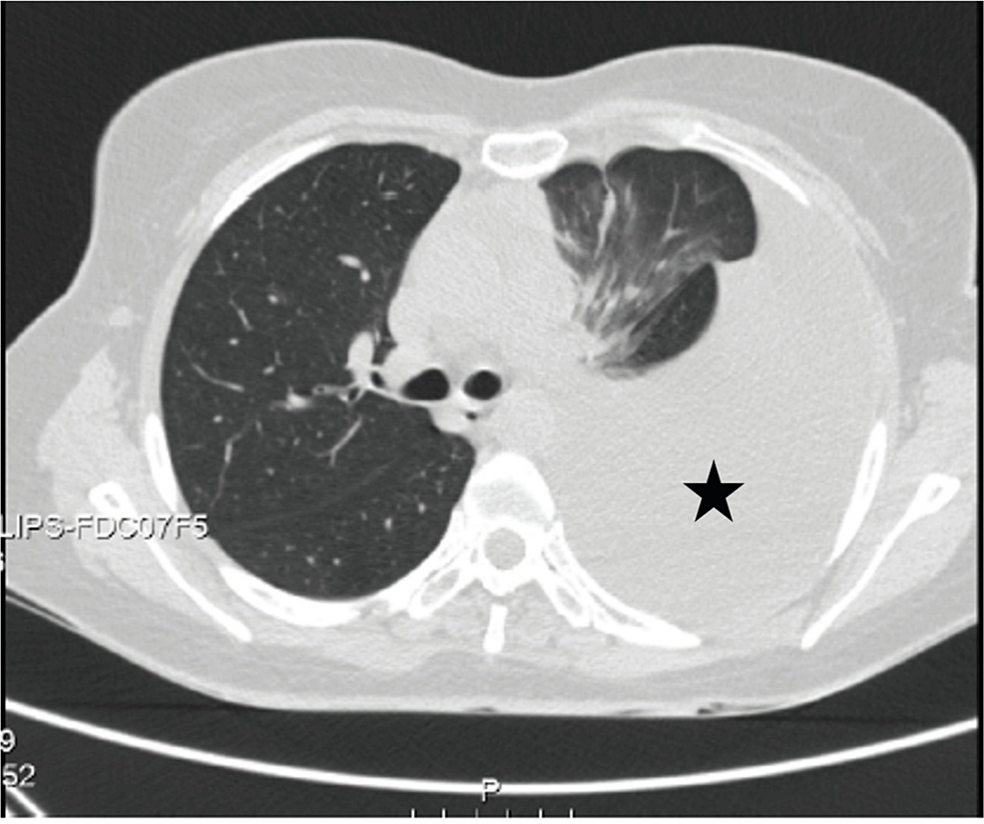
Left pleural effusion (chylothorax) (star) (CT axial lung window).
